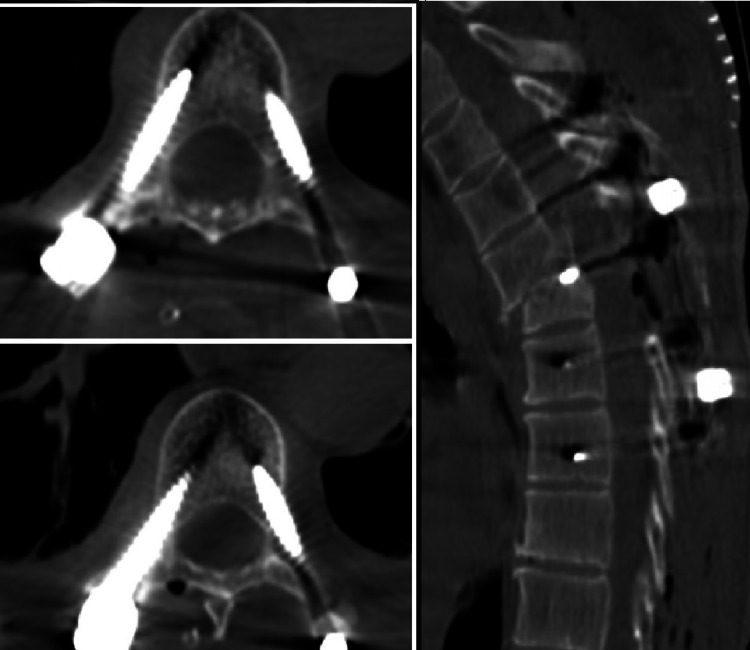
Postoperative CT image of a male patient operated with 3D modeling technique.
